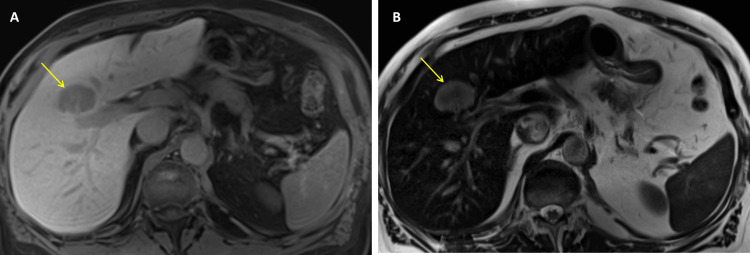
MRI and diagnosis of intrahepatic cholangiocarcinoma Magnetic resonance imaging (MRI – A, axial T1; B, axial T2) after the initial LuPSMA cycle showed an interval increase in size of an isolated right hepatic lobe mass (yellow arrow), now measuring 3.1 × 4.1 cm (previously 1.3 cm on prior MRI, not shown), compatible with neoplasm such as hepatocellular carcinoma or possible metastasis. No other suspicious hepatic lesions were identified.
